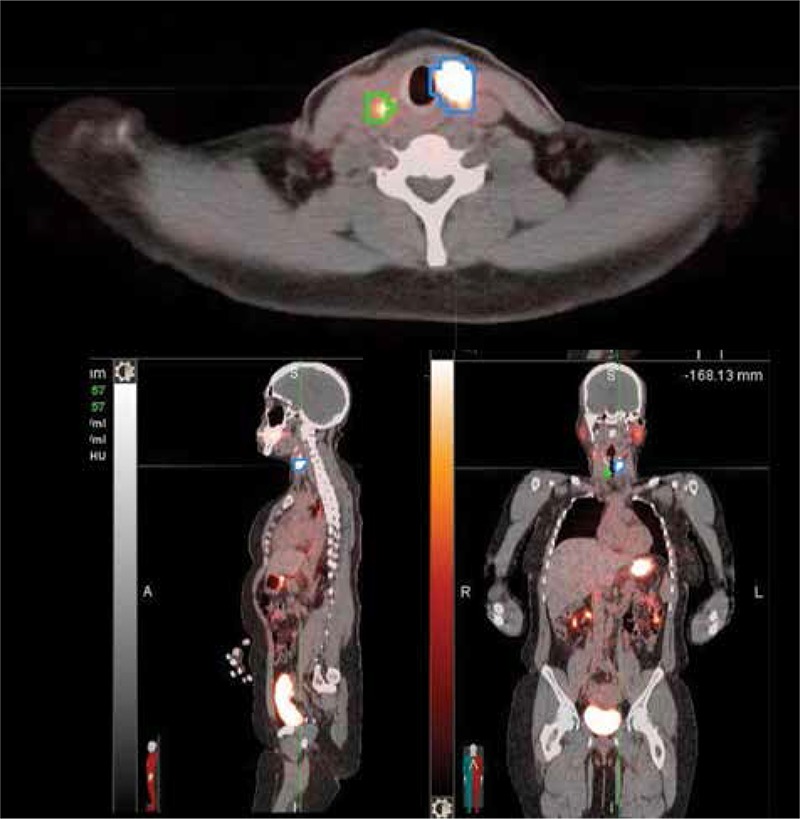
I-124 positron emission tomography/computed tomography image through the level of the thyroid bed shows regions of interest drawn around a thyroid remnant (blue) and a metastatic right level 3 lymph node (green). The remnant is not measurable by ultrasound or computed tomography. There is overestimation of functional volume (correctible) due to voxel saturation phenomenon
